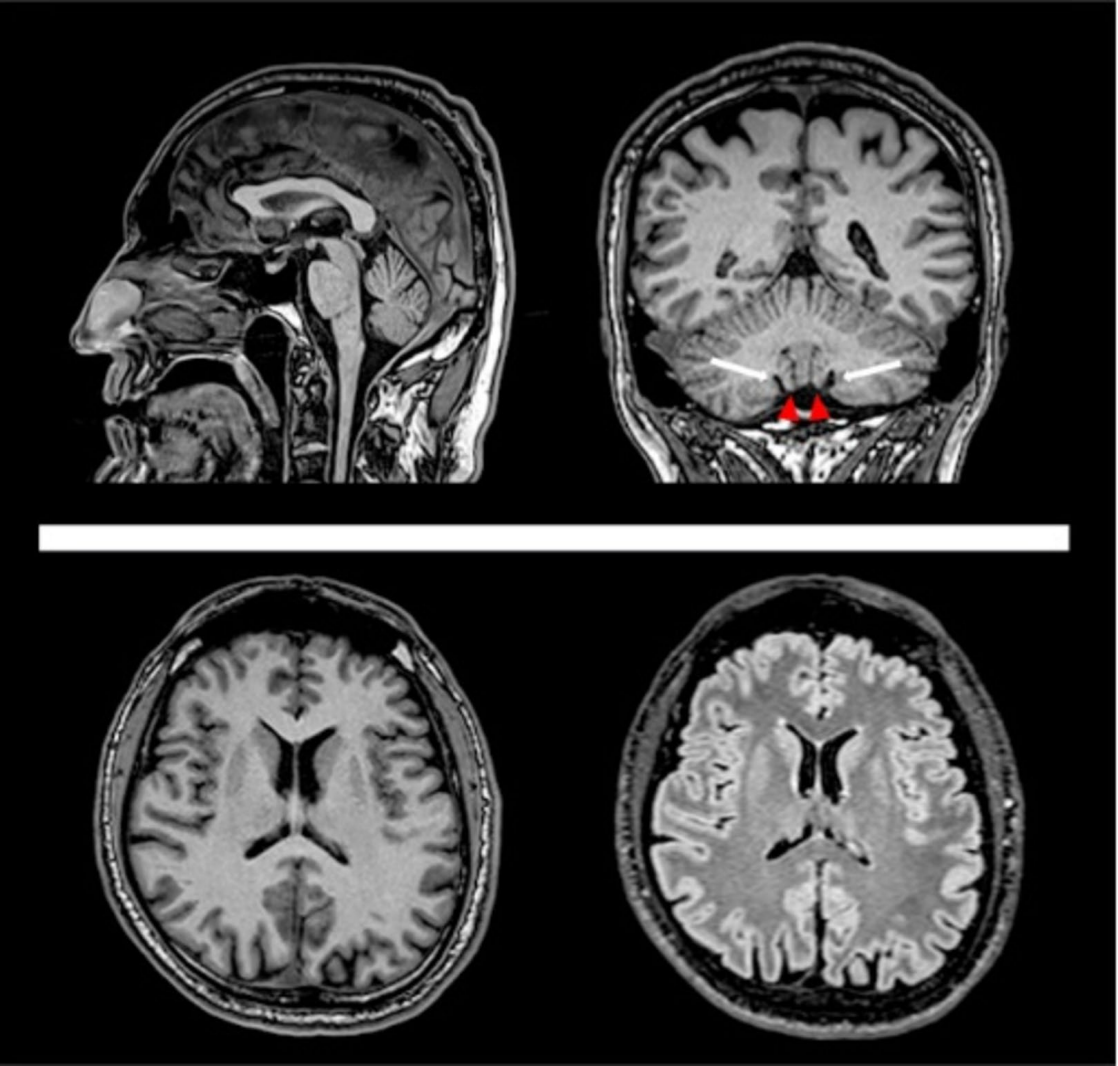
Brain MRI. Representative images of the brain magnetic resonance imaging (MRI) scan in the index case (II-5). In the upper row, a sagittal slice of a 3D volumetric T1-weighted image on the left shows the absence of significant brainstem or vermian atrophy, coupled to a preserved volume of the corpus callosum. The corresponding coronal multi-planar reconstruction on the right shows the occurrence of a selective atrophy of the cerebellar tonsils (red arrowheads), confirmed by a selective enlargement of the retrotonsillar fissure (white arrows) in the context of a preserved cerebellar volume. In the lower row, two axial multi-planar reconstructions of a 3D volumetric T1-weighted image (left) and a 3D Fluid Attenuated Inversion Recovery (FLAIR) sequence (right) show the absence of significant brain atrophy and signal changes, respectively
